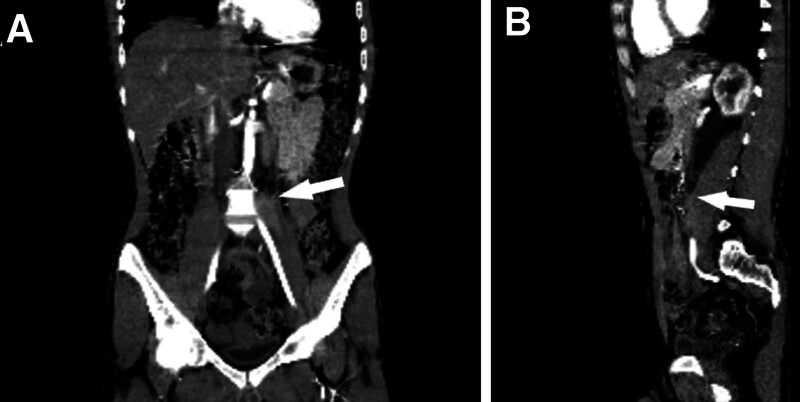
Contrast-enhanced computed tomography in the coronal section (A) and sagittal section (B) revealed a lesion obstructing the ureter at the level of the fourth vertebra, resulting in ureteral dilatation proximal to the obstruction (indicated by arrows).
